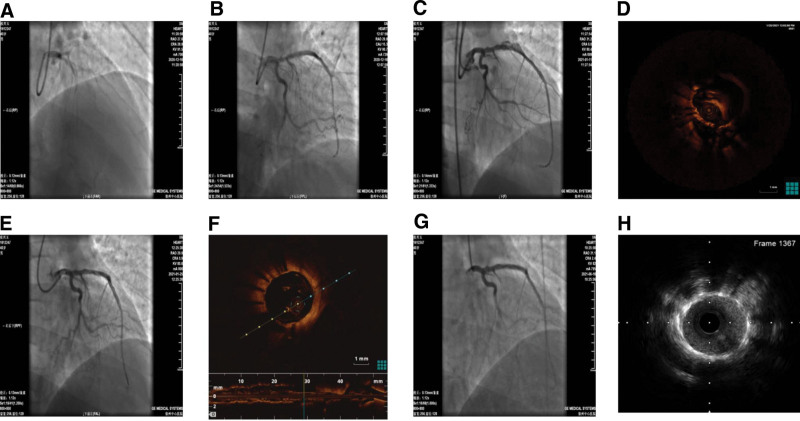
Images obtained before and after stent implantation. (A), CAG showed total occlusion of the middle segment of the LAD after the origin of D1. (B), CAG immediately after PCI. (C), CAG at 5 weeks after PCI revealed a fusiform aneurysm of the middle segment of the LAD. (D), OCT demonstrated interruption of the 3-layered structure of the wall of the middle segment of the stented LAD and outward protrusion of the adventitia. (E), CAG immediately after implantation of a coated stent in the middle segment of the LAD. (F), OCT showed complete closure of the aneurysm body and good adherence of the coated stent to the vascular wall. (G), CAG performed 5 months after implantation of the coated stent demonstrated a patent LAD with no evidence of restenosis in the stented middle segment. (H), IVUS performed 5 months after implantation of the coated stent showed no evidence of intimal hyperplasia or in-stent thrombogenesis. CAG = coronary arteriography, IVUS = intravascular ultrasound, LAD = left anterior descending artery, OCT = optical coherence tomography, PCI = percutaneous coronary intervention.
